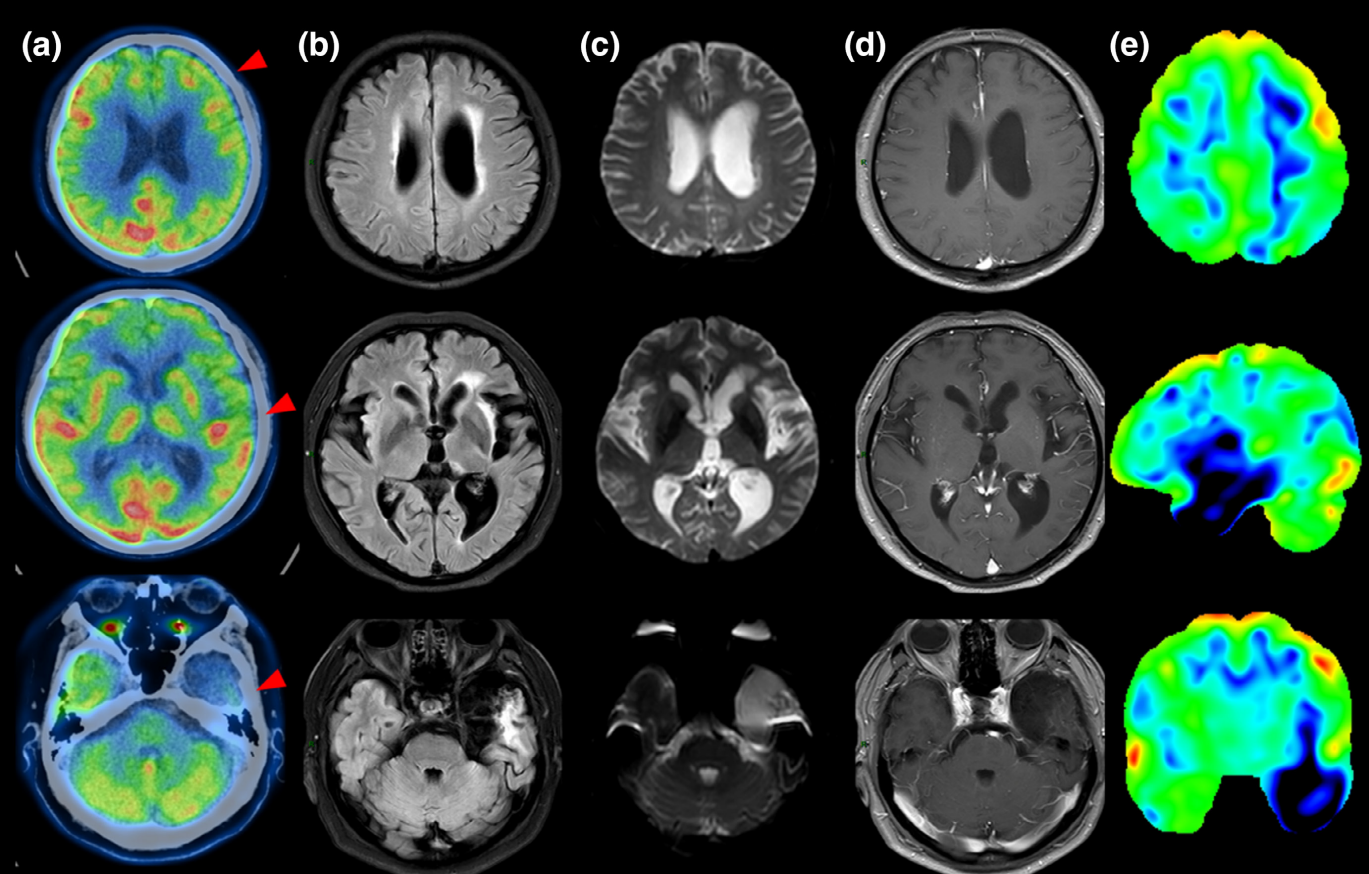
A typical case of the PET scan time for more than 3 months from the onset of symptoms (pat. 3 had intermittent fever with nausea, dizziness, headache, and cognitive impairment). FDG‐PET of 109 days revealed hypometabolism in the in the left middle frontal and left temporal lobe (A). T2‐FLAIR showed hyperintensities of axial MRI in the left temporal lobe, hippocampus, and insular lobe in 57 days (B). The left temporal lobe showed hyperintensities in DWI (C). There was no enhancement in mentioned above areas of contrast‐enhanced MRI (D). The FDG statistical deviation images analysis based on the *z*‐score (E).
